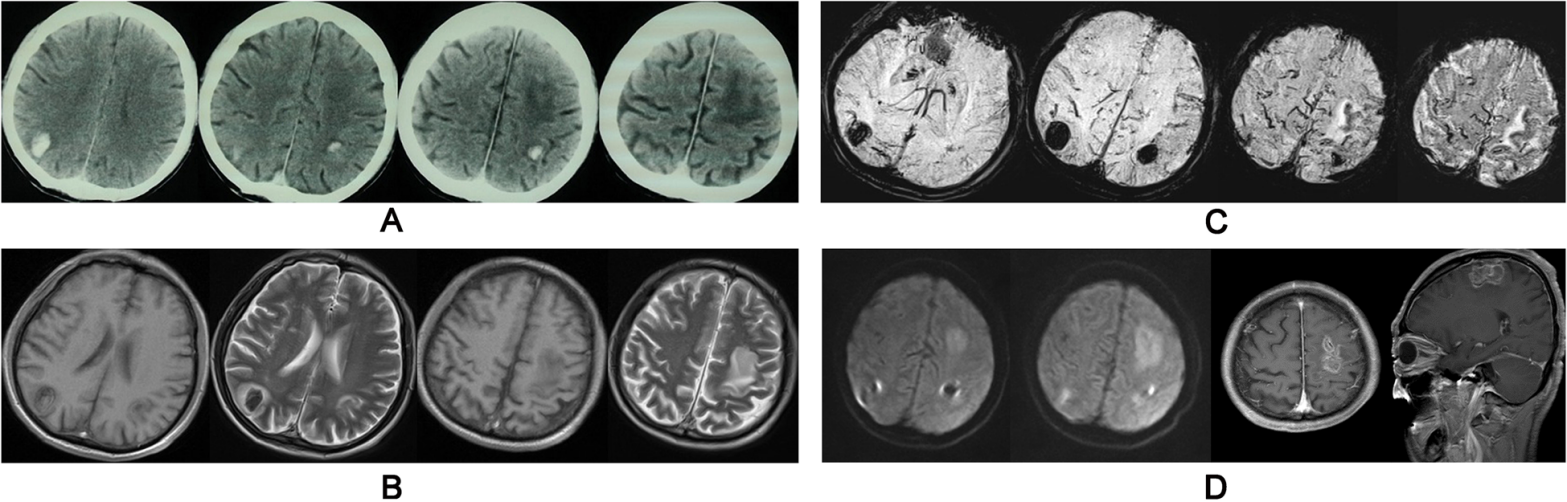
Lesions displayed on the head CT and MRI.Multiple hemorrhages located in the bilateral parietal lobes were revealed, as well as an infarction with hemorrhagic transformation in the left frontal lobe on the head plain CT (a), T1WI/T2WI (b), SWI (c), DWI (d, first two images), and gadolinium-enhanced MRI (d, last two images)
